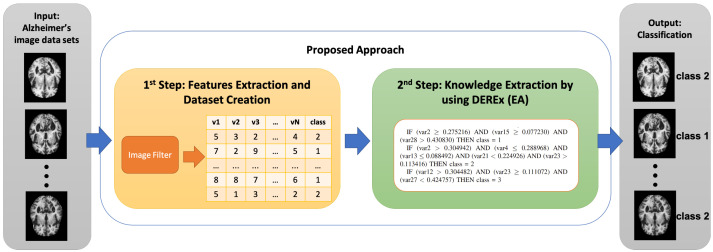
The approach. The left part of the image contains the filter (1st step), whereas the right part contains the classifier (2nd step) based on an Evolutionary Algorithm (EA). This latter also extracts explicit knowledge. MRI images contained in this figure are taken from the original Alzheimer’s data set [53] available on Kaggle.
